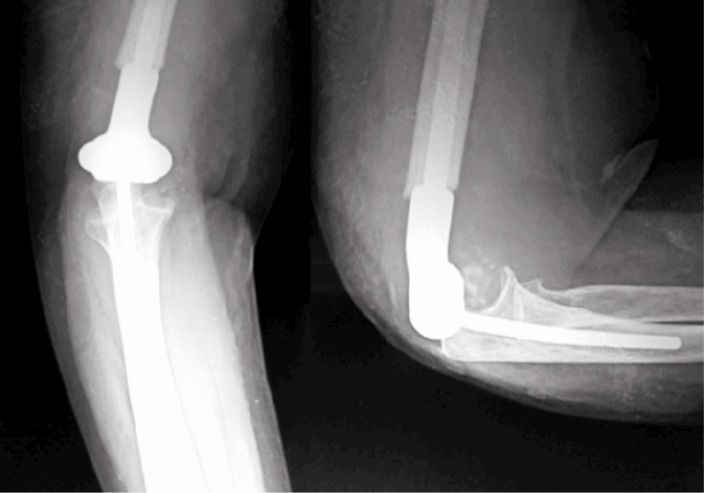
Post-operative plain radiograph showing good position of prosthesis and no evidence of recurrence or loosening at 6 months.
